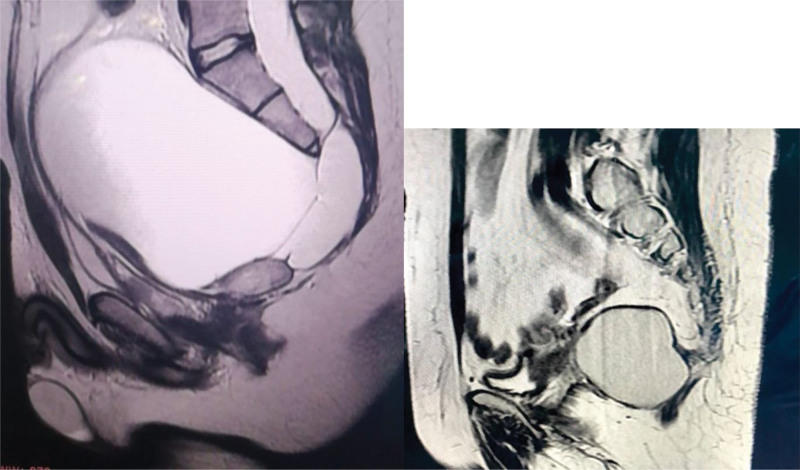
T2-weighted MRI showing a well-defined cystic retrorectal lesion (arrow) located between the rectum and the sacrum. MRI = magnetic resonance imaging.
